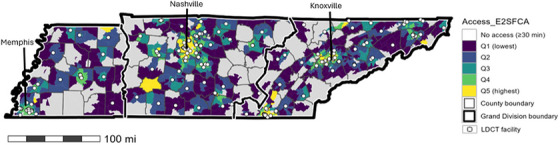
LDCT accessibility across Tennessee (E2SFCA, 30‐min, three band decay). E2SFCA, enhanced two‐step floating catchment area; LDCT, low‐dose computed tomography.
